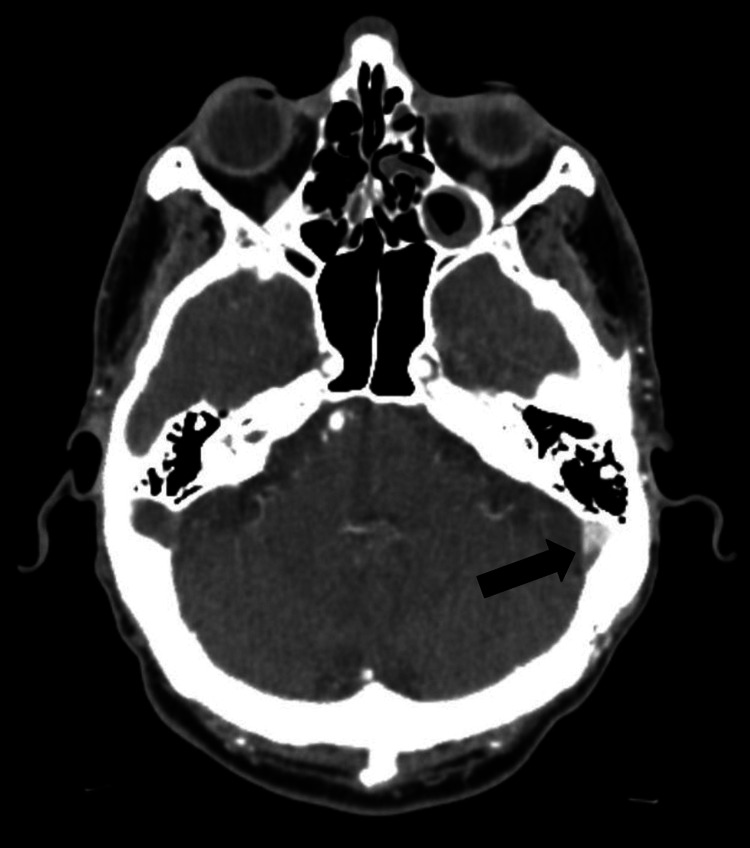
CT venogram showing left transverse sinus thrombosis (black arrow)
